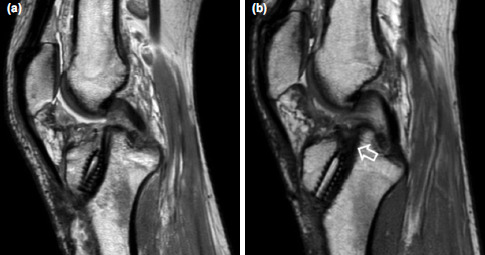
MRI images of tibial tunnel with graft in situ of a participant. (a) Pre-ESWT with no incorporation and, (b) was the post-ESWT that demonstrates partial incorporation (open arrow).
